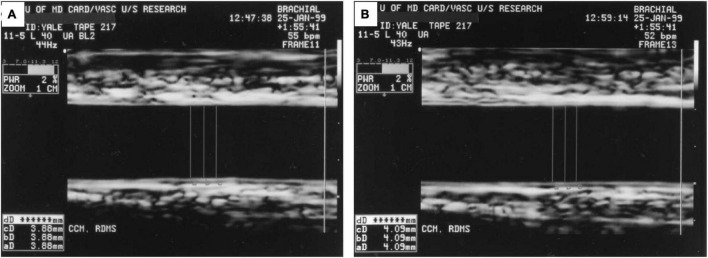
Ultrasound Images Demonstrating Brachial Flow-Mediated Dilatation. (A) Shows the brachial artery at rest with arterial diameter of 3.88 mm. (B) Shows the artery 1 min after hyperemic stimulus with arterial diameter of 4.09 mm. Figure reproduced with permission from Corretti et al. (9) copyright JACC (Elsevier).
